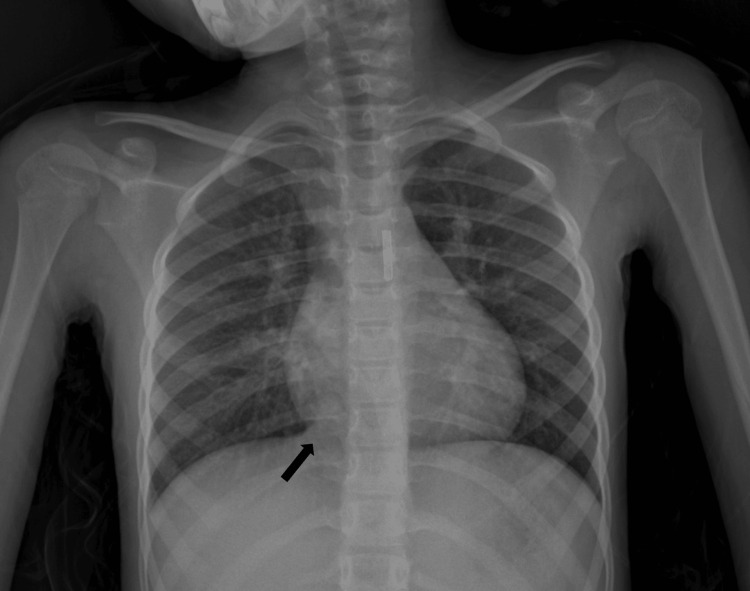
Posteroanterior chest radiograph Right paracardiac consolidation (black arrow) along the lateral aspect of the right heart border without obliteration of the cardiac silhouette is noted.
